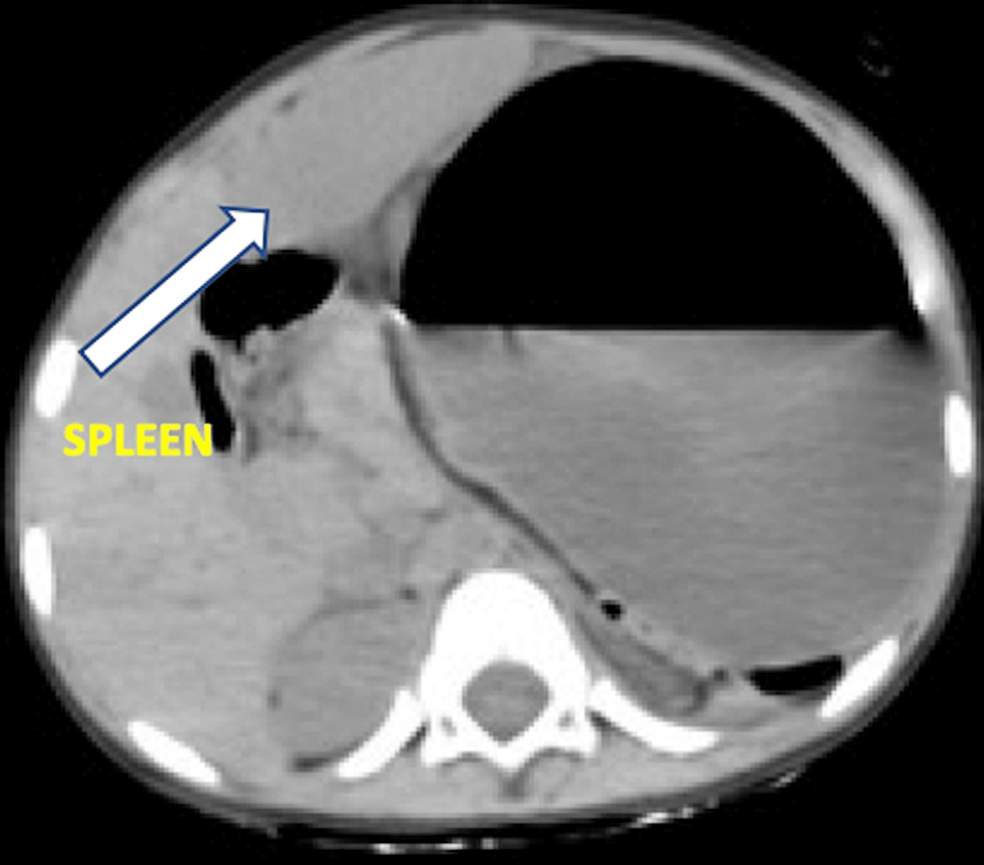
Non-contrast CT abdomen axial section showing paramidline right-sided spleen with overdistended stomach.
